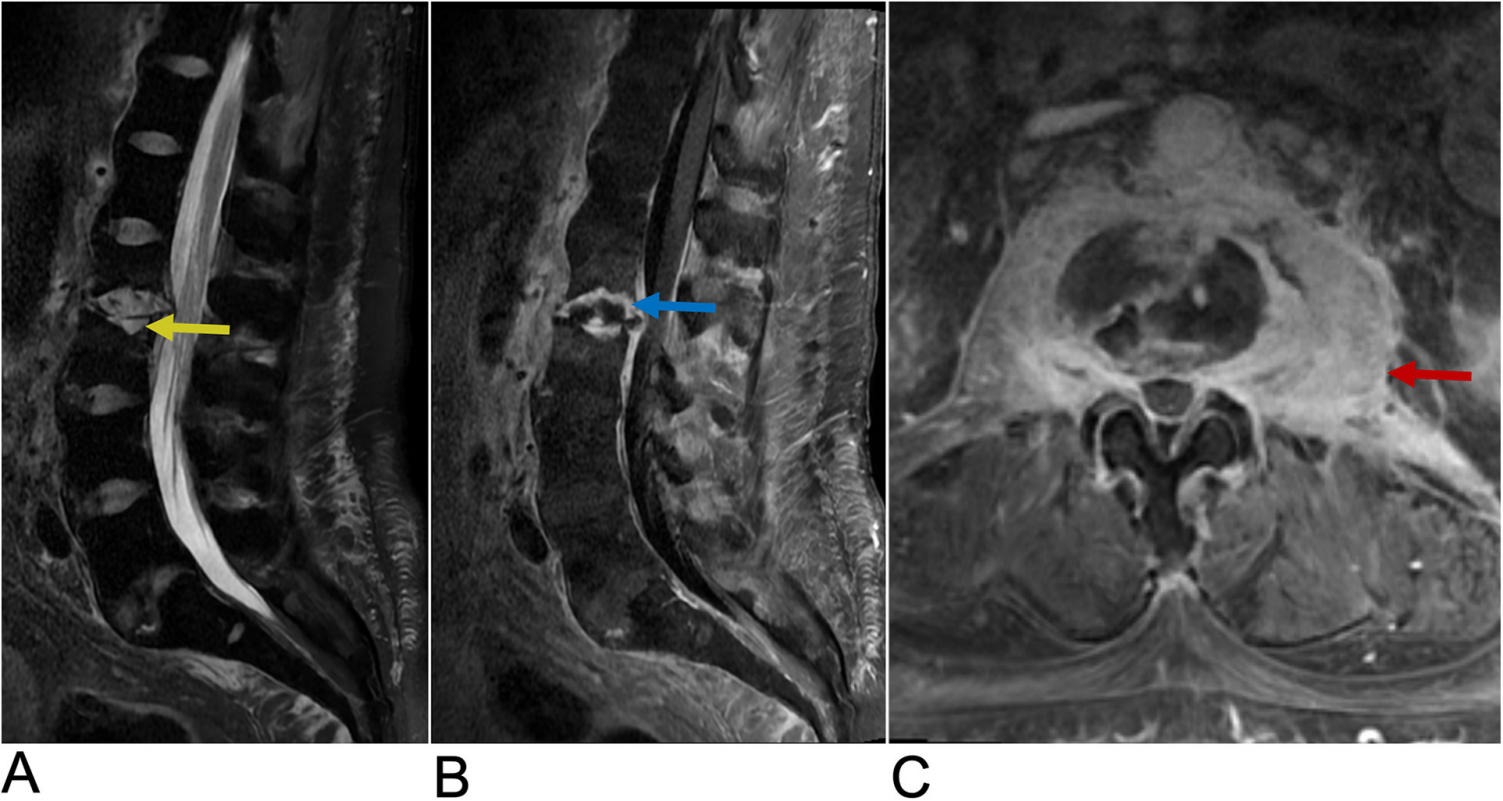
Discitis-Osteomyelitis. Sagittal T2 (A), Sagittal T1 + C (B), and axial T1 + C (C) MRI of the lumbar spine demonstrating acute discitis osteomyelitis involving the L2–L3 level with endplate erosive changes (yellow arrow), post-contrast enhancement (blue arrow), and trace fluid in the intervertebral disc space. There is posterior ventral epidural extension of the phlegmonous changes and bilateral paravertebral extension of the enhancement involving the psoas muscles bilaterally (red arrow).
